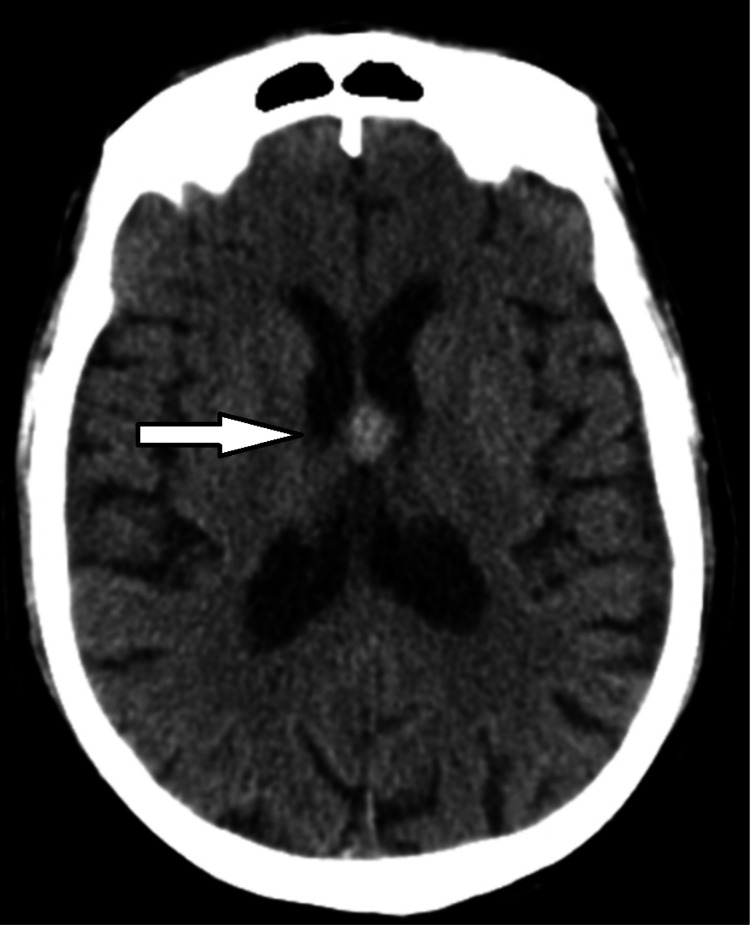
Cranial computed tomography (CT) showing a hyperdense lesion, localized to the inferior pole of the septum pellucidum, suggestive of recent hemorrhage
